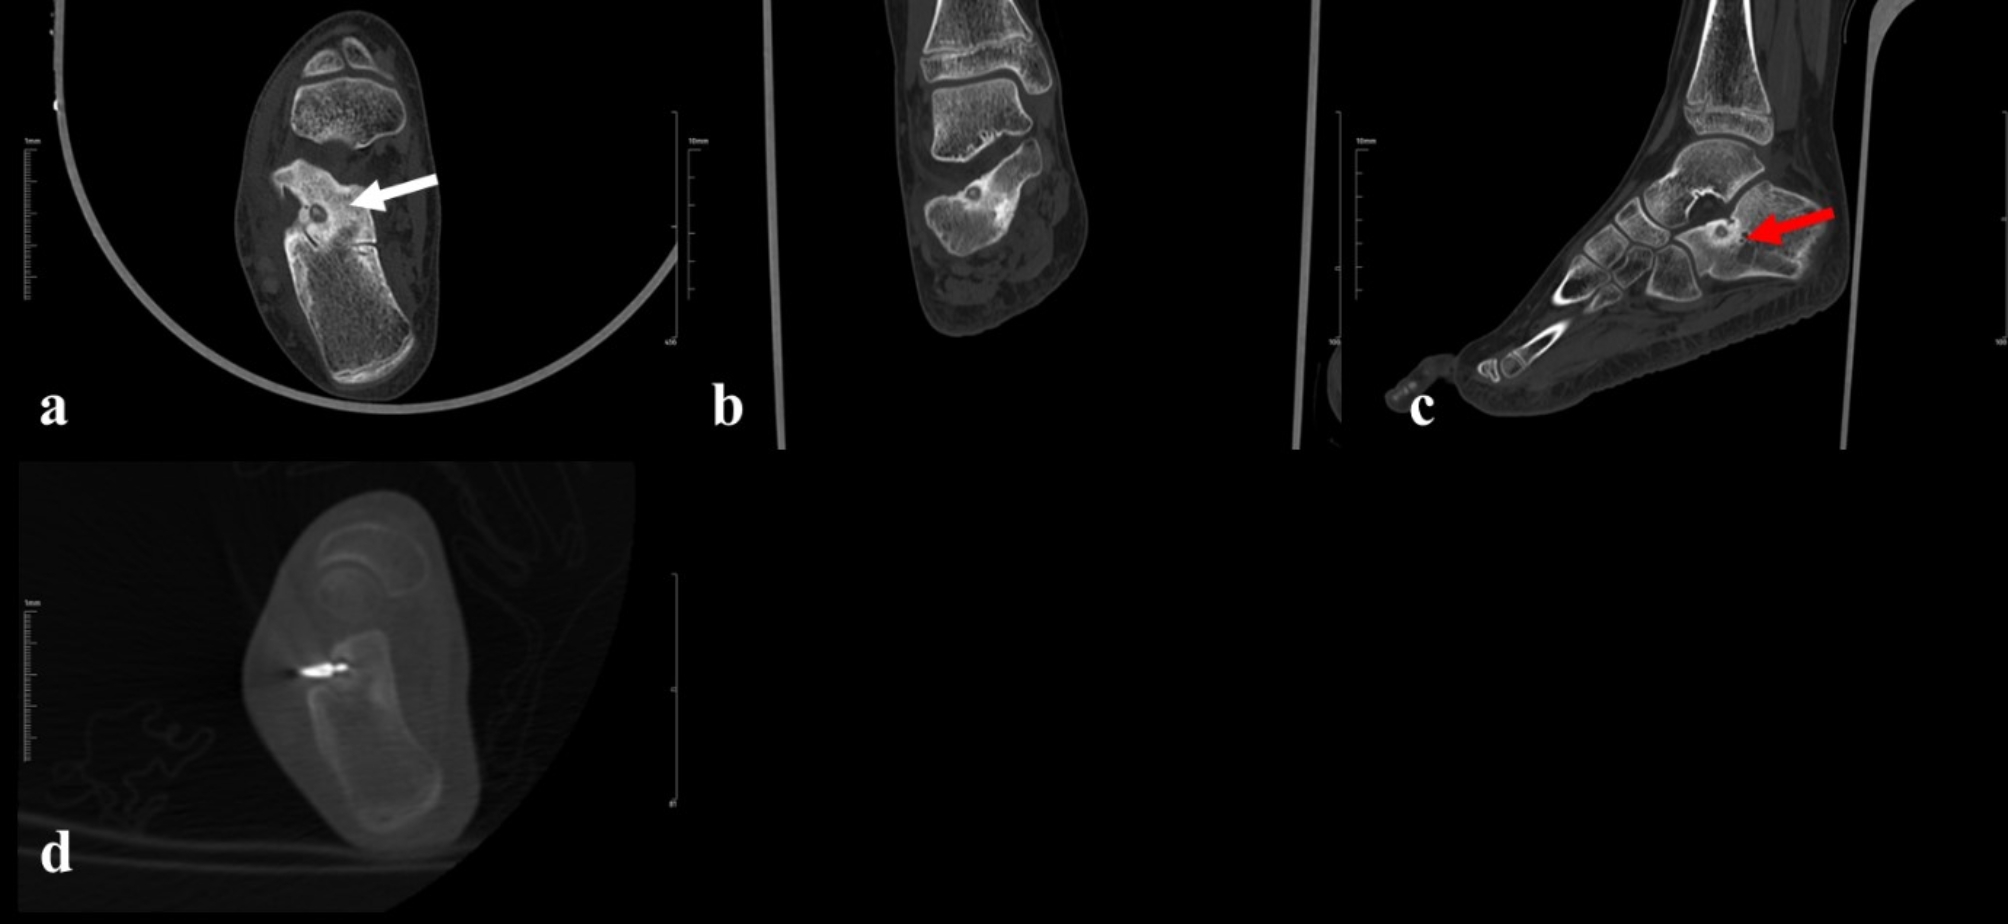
In an 11-year-old female patient admitted to outpatient clinic with feet pain, peripherally sclerotic and centrally hypodense lesion located in the calcaneus is shown on CT images (red arrow). a.) in pre-op axial sections, b.) in pre-op coronal sections, c.) in pre-op sagittal sections the nidus diameter was 7 mm, and the periosteal reaction (White arrow) thickness was 4 mm, d.) and in the image during processing a bone penetration needle (11 F; APRO Korea Inc., Gunpo, Korea) is inserted into the lesion nidus. Afterwards, the burning process is applied at 100 degrees for 4–6 min. The pre-procedural pain score was 10, the pain score decreased to 1 point in the 1st week and 3rd month after the procedure. The patient uses NSAIDs 7 times a week (1 daily) before the procedure, she does not use any NSAIDs after the procedure
